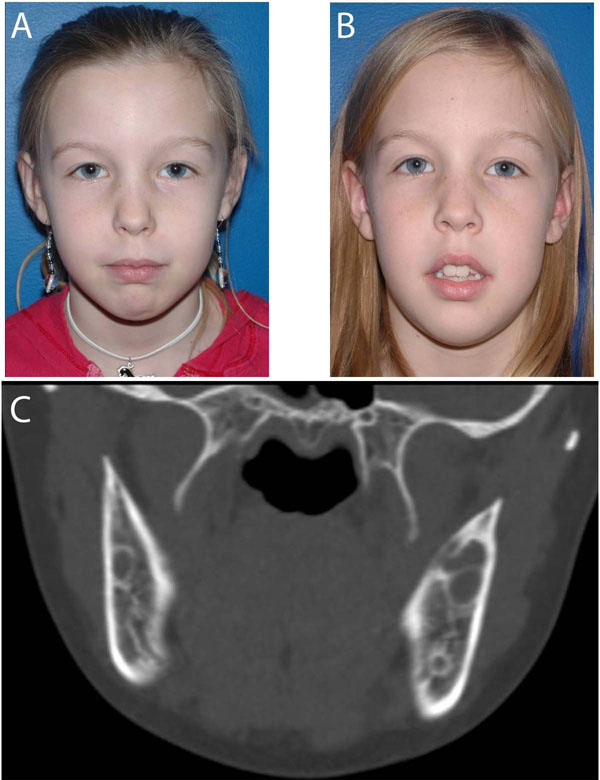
A. Photograph of a 10 year old girl with bilateral cheek and jaw swelling. Cherubism was confirmed with genetic testing that was positive for the SH3BP2 gene. The patient had genetic counseling and was followed longitudinally. B. Patient one year later with no progression of facial swelling. C. CT scan at initial presentation showed typical bilateral lytic lesions in the mandible that remained unchanged at follow ups.
